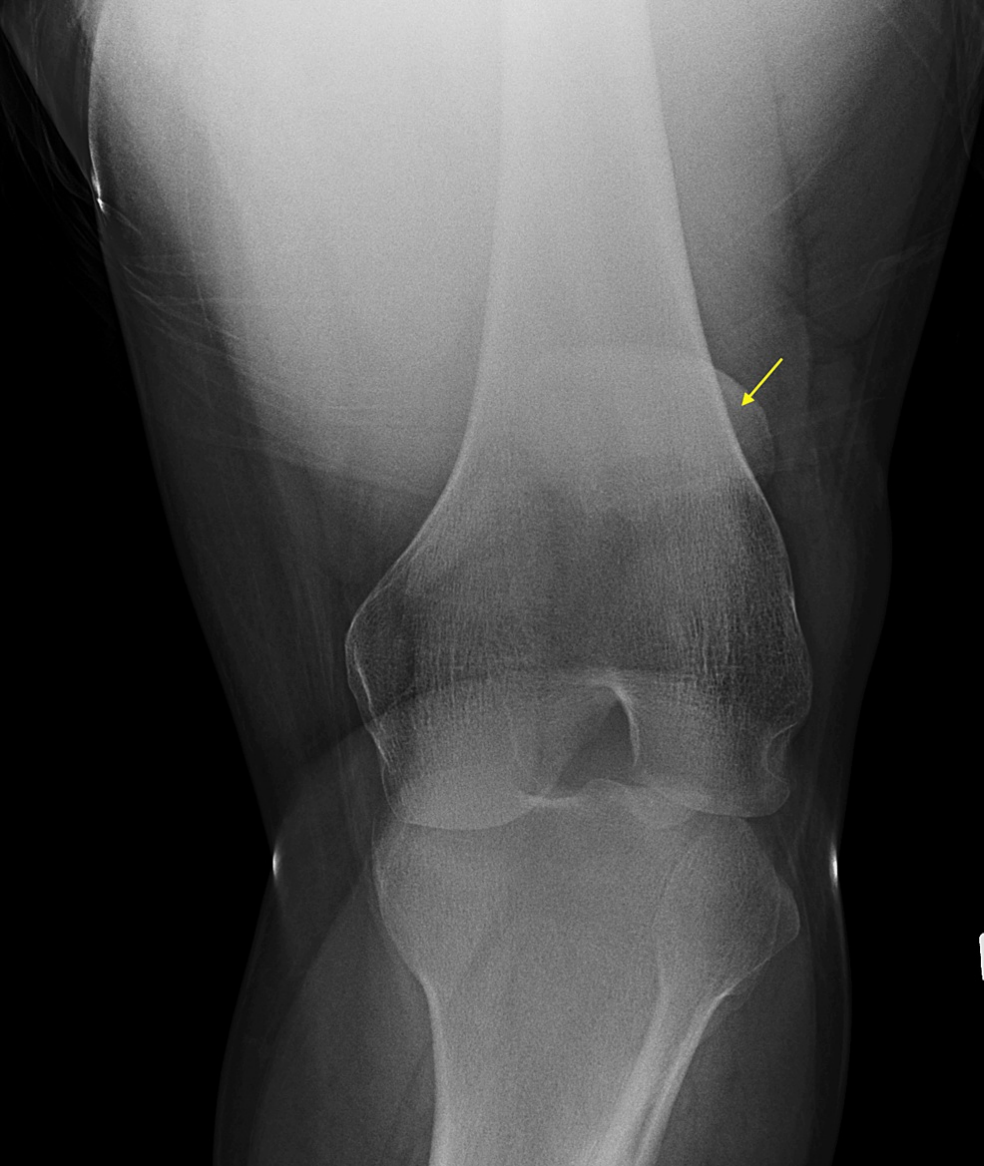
Radiograph depicting an anteroposterior view of the patella (arrow)
